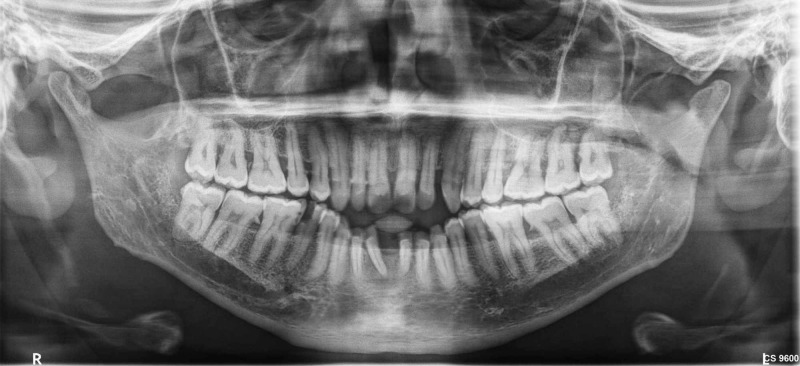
Orthopantomogram of a chronic periodontitis patient
